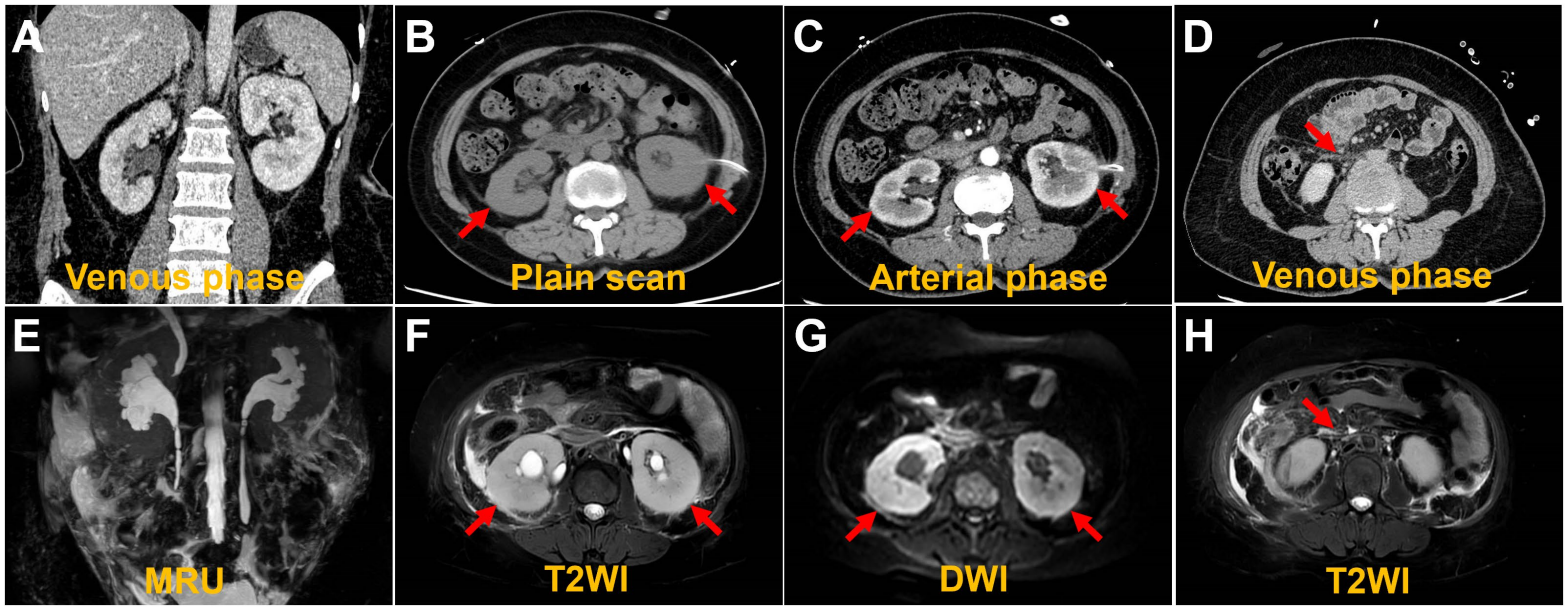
Computed tomography images and magnetic resonance images. The coronal image shows bilateral hydronephrosis and enlarged kidneys (A,E). The axial images show diffuse lesions in the renal parenchyma, with no obvious masses detected (B,C,F,G). The axial image shows retroperitoneal multiple exudation (D,H).
